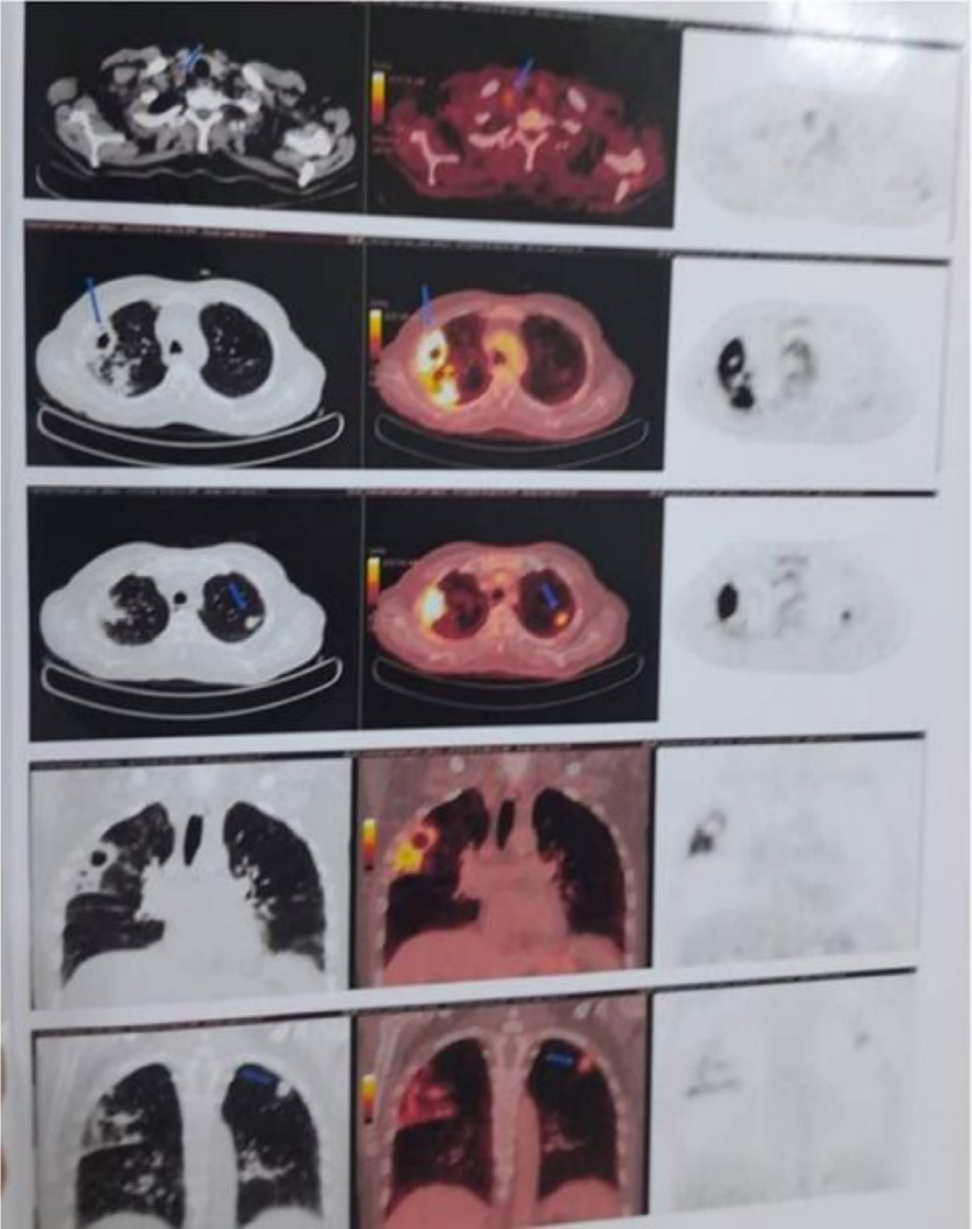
PET CT scan findings are suggestive of a metabolically active cavitary lesion in the right upper lobe, along with bilateral pulmonary nodules. Low‐grade metabolically active right, lower cervical, right mediastinal, and right hilar lymph nodes on the right side were noted. No evidence of any metabolically active disease observed elsewhere.
